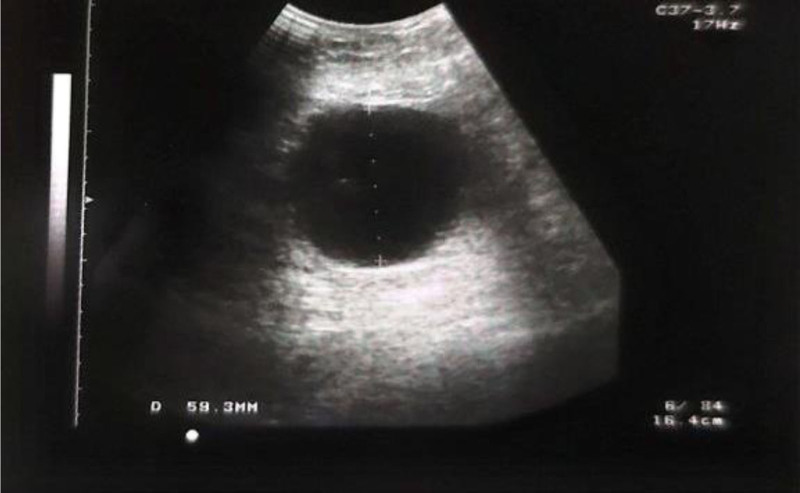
Ultrasound view of the complex mass/cyst.
